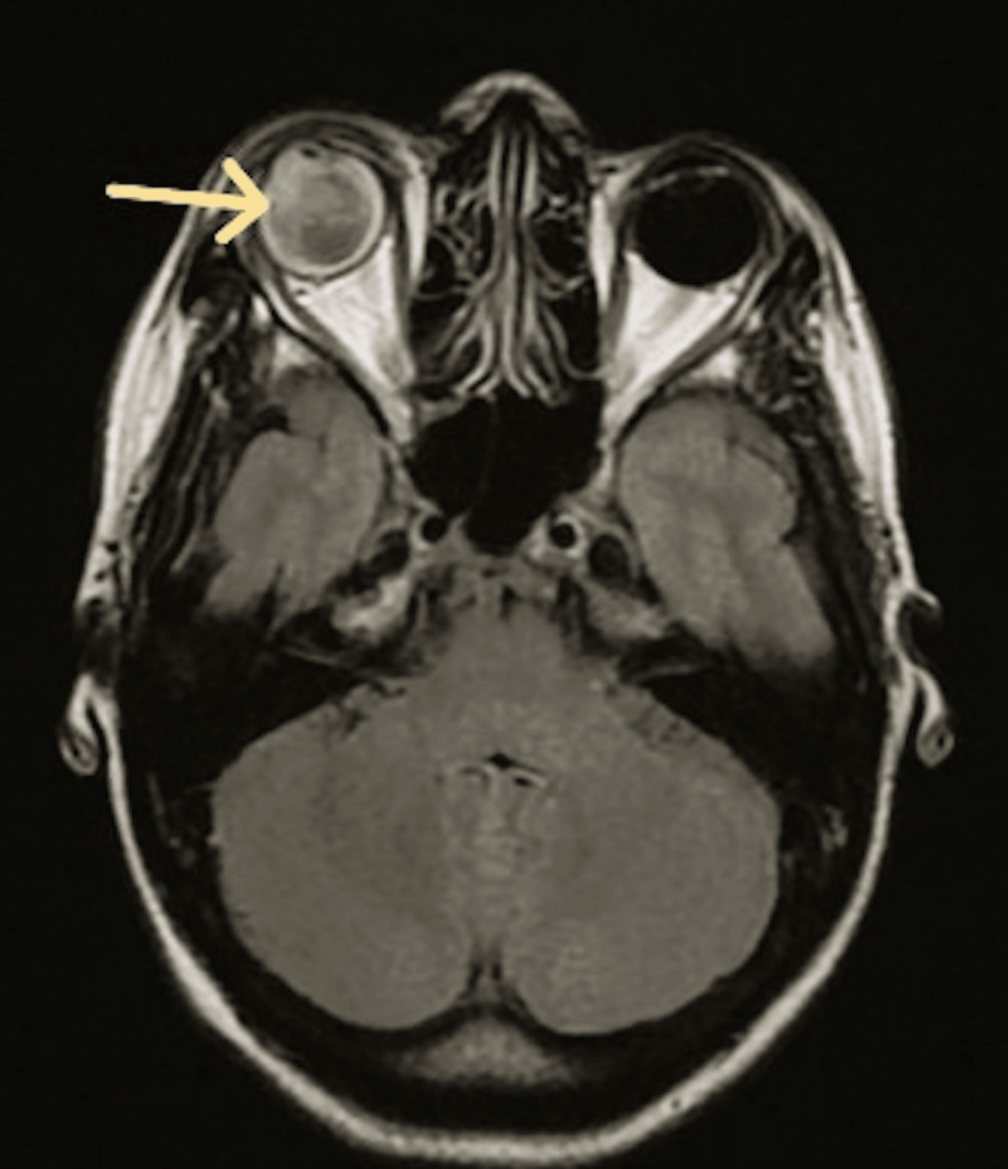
Cranial MRI revealing a vitreous hemorrhage with an intraocular mass in the anteroinferior quadrant, demonstrating marked periorbital fat infiltration.
